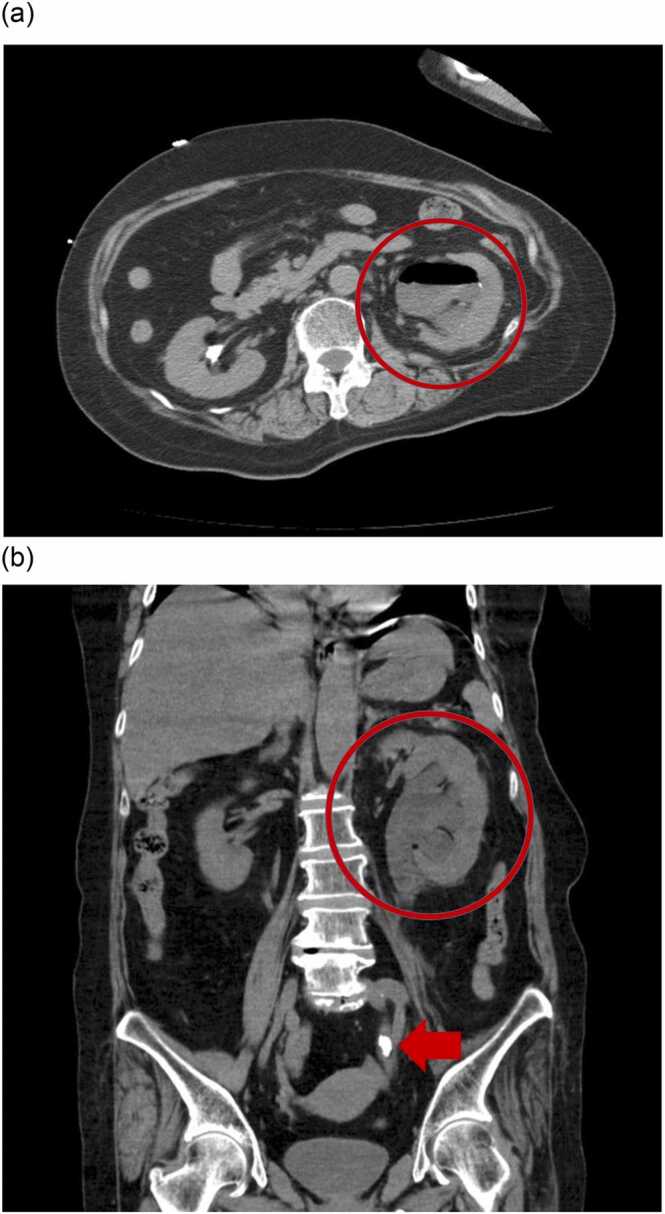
Non enhanced abdominal CT showed a stone in the left ureter and left hydronephrosis with gas images in the left renal pelvis. A) Axial view. B) Coronal view. *Note*: Circles indicate hydronephrosis; arrows indicate stone.
